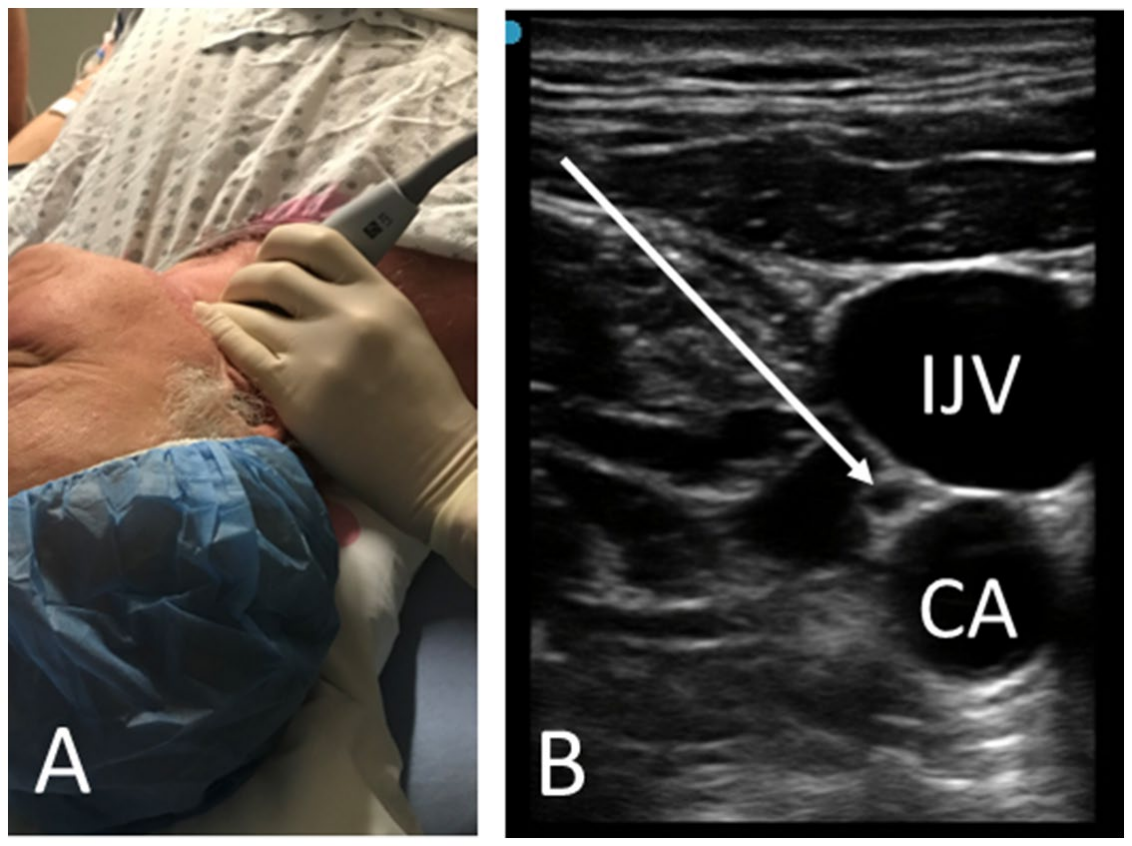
Ultrasound technique (A) and image (B) showing vagus nerve and direction of needle (arrow), carotid artery (CA) and internal jugular vein (IJV).
